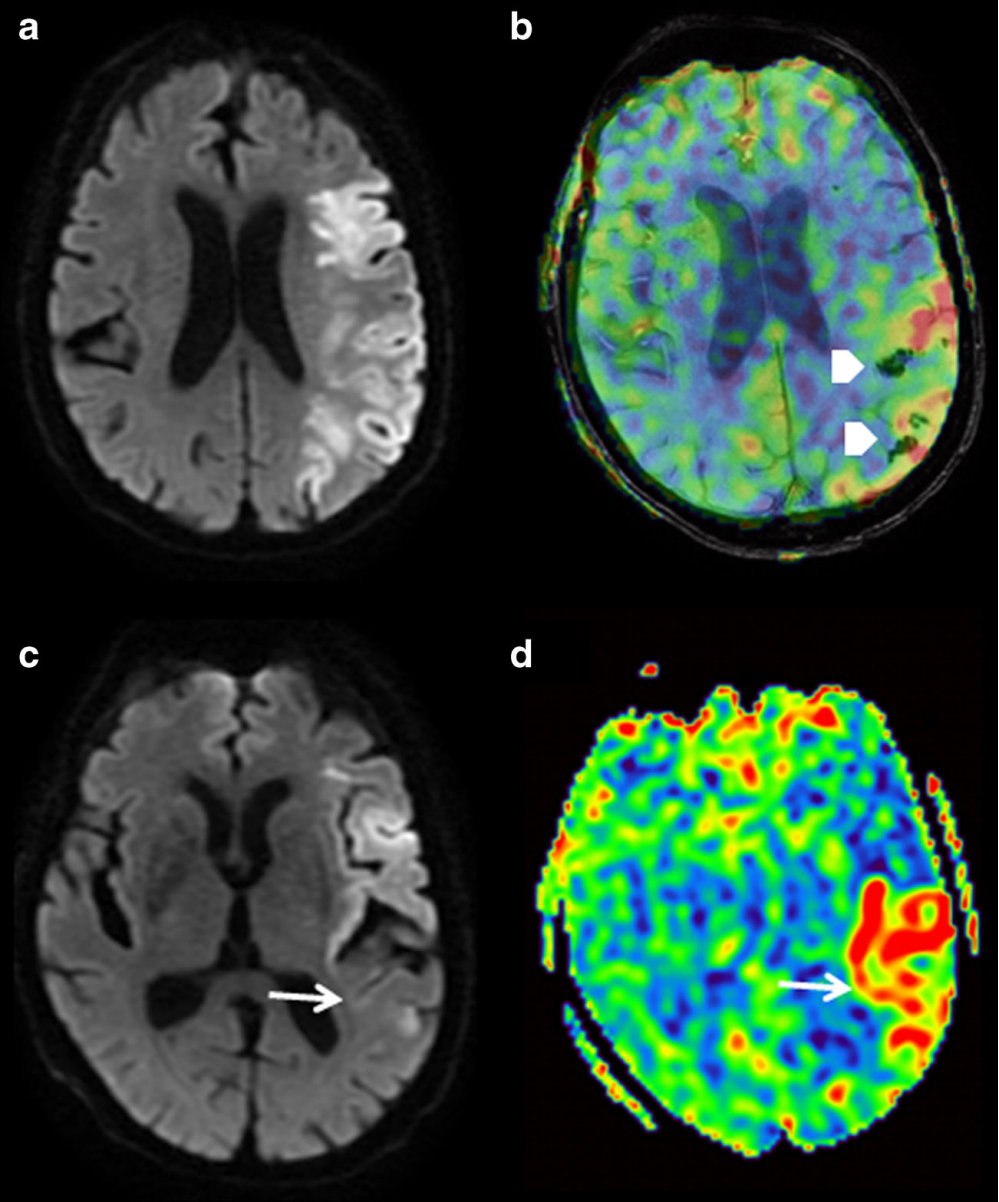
Control image 24 h after intravenous thrombolysis in a patient seen in an emergency setting for a superficial left sylvian ischaemia with favourable clinical outcome. DWI (a and c) SWI/ASL fusion (b), and ASL (d) sequences. a Left superficial sylvian acute ischaemic lesion. b Zones of hypointense haemorrhagic transformations on the SWI sequence superimpose with the hyperperfusion zones on the ASL (*arrowheads*). c and d Anterior sylvian involvement with partial recovery on the DWI images of the posterior portion corresponding to the zone of hyperperfusion (*white arrows*)
